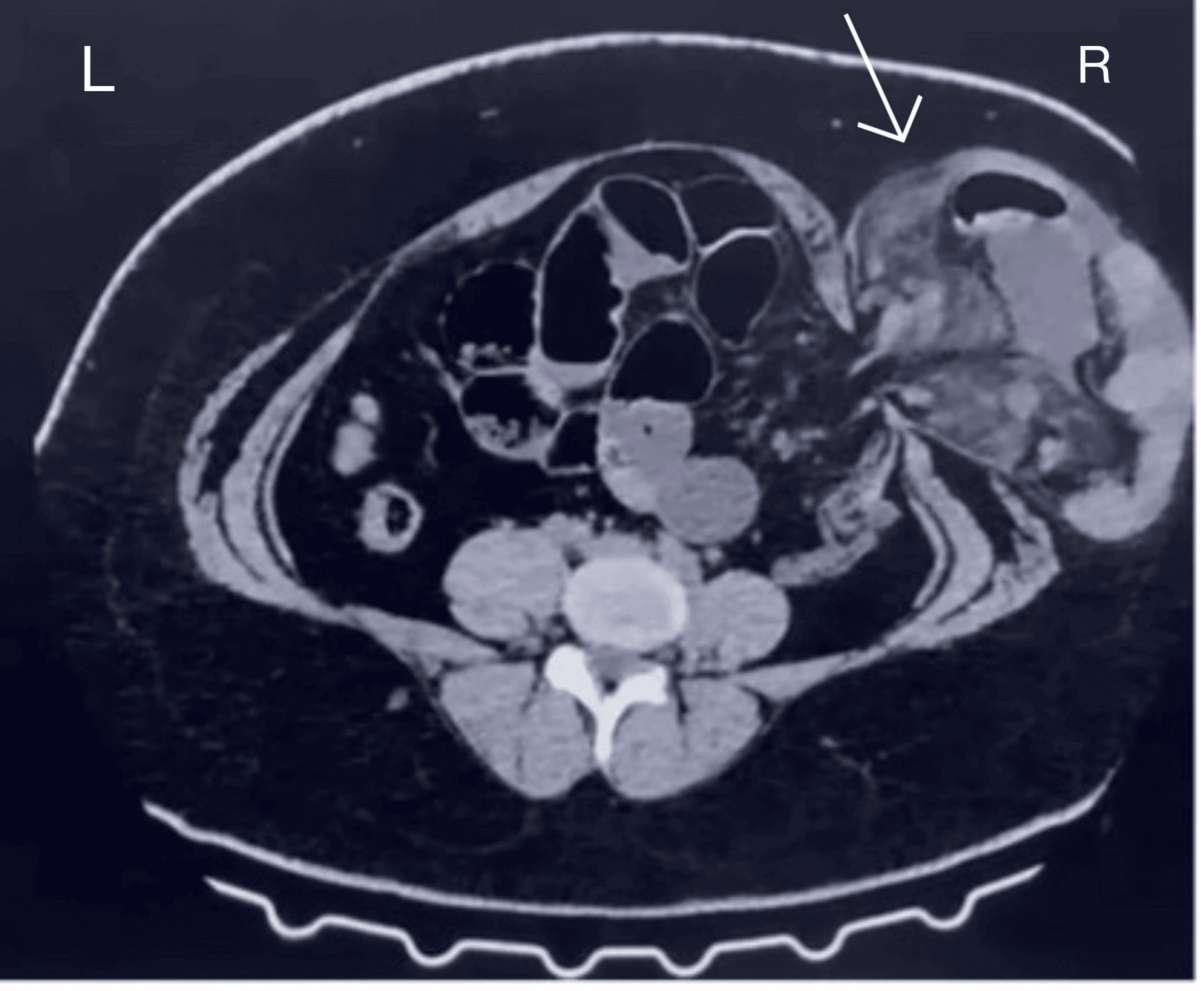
Contrast-enhanced CT abdomen showing herniation of bowel loops with omentum in the right lumbar region (white arrow).
